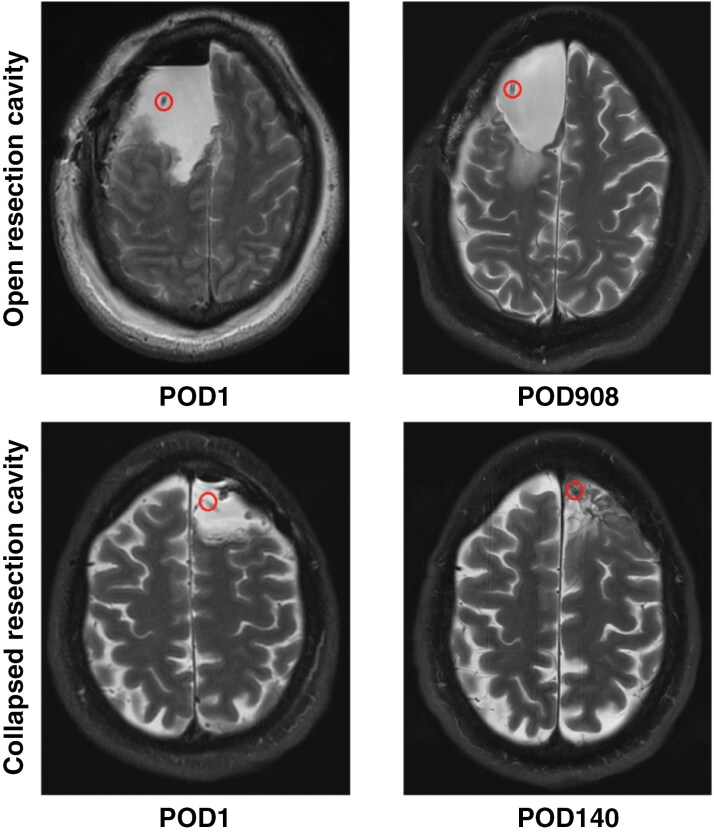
Ommaya reservoir catheters in the resection cavity. The location of the ventricular catheters attached to Ommaya reservoirs over time is shown in open (left) versus collapsed (right) resection cavities from two patients on T2-weighted MRI scans. The catheter tip is circled.
